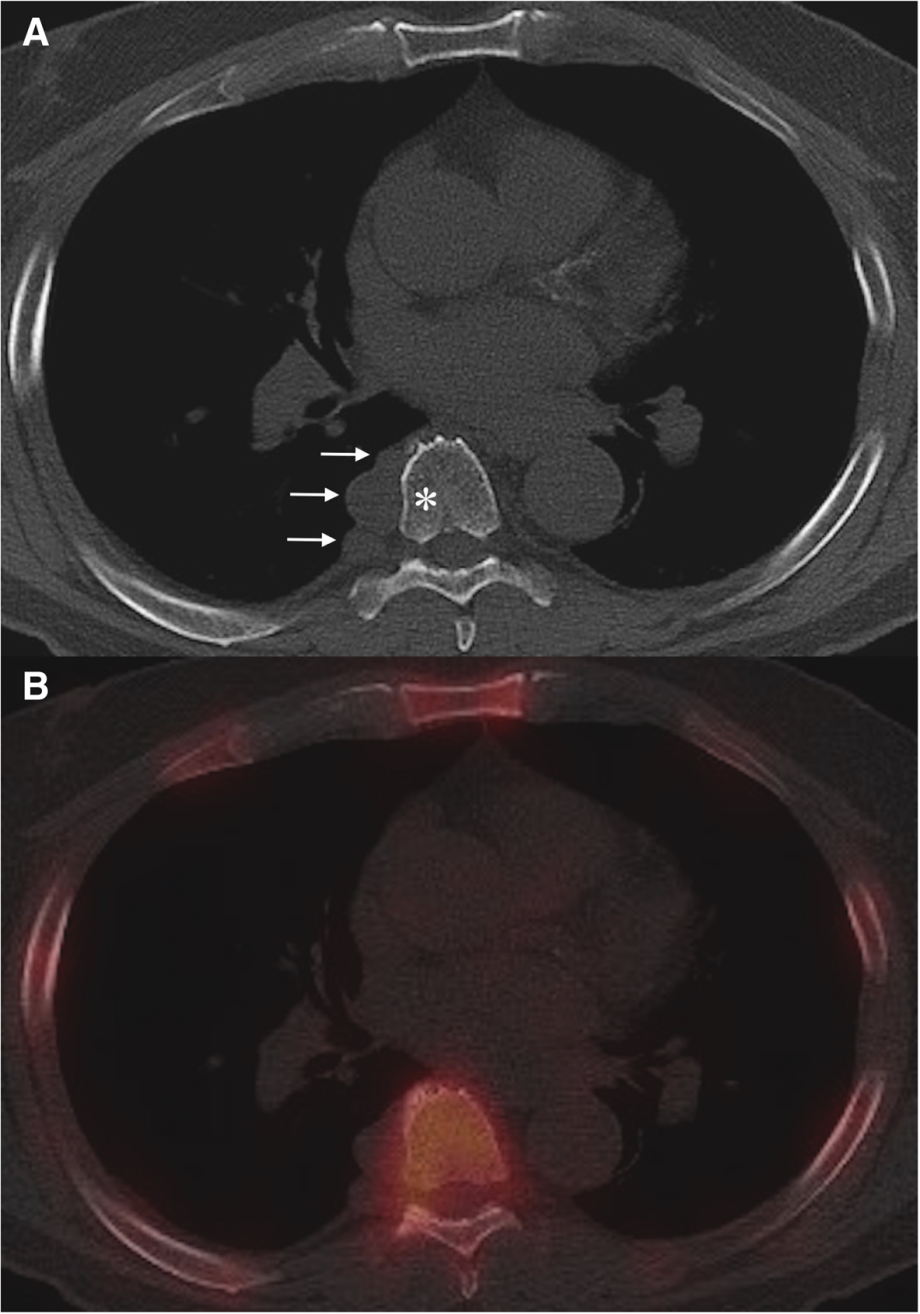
SPECT/CT 99mTc-MDP bone scan. a Axial CT image shows three extrapleural nodules (arrows) adjacent to the T7 vertebral body, which has a notched appearance (*). b Axial fused SPECT/CT image demonstrates no evidence of proximate bony metastatic disease
